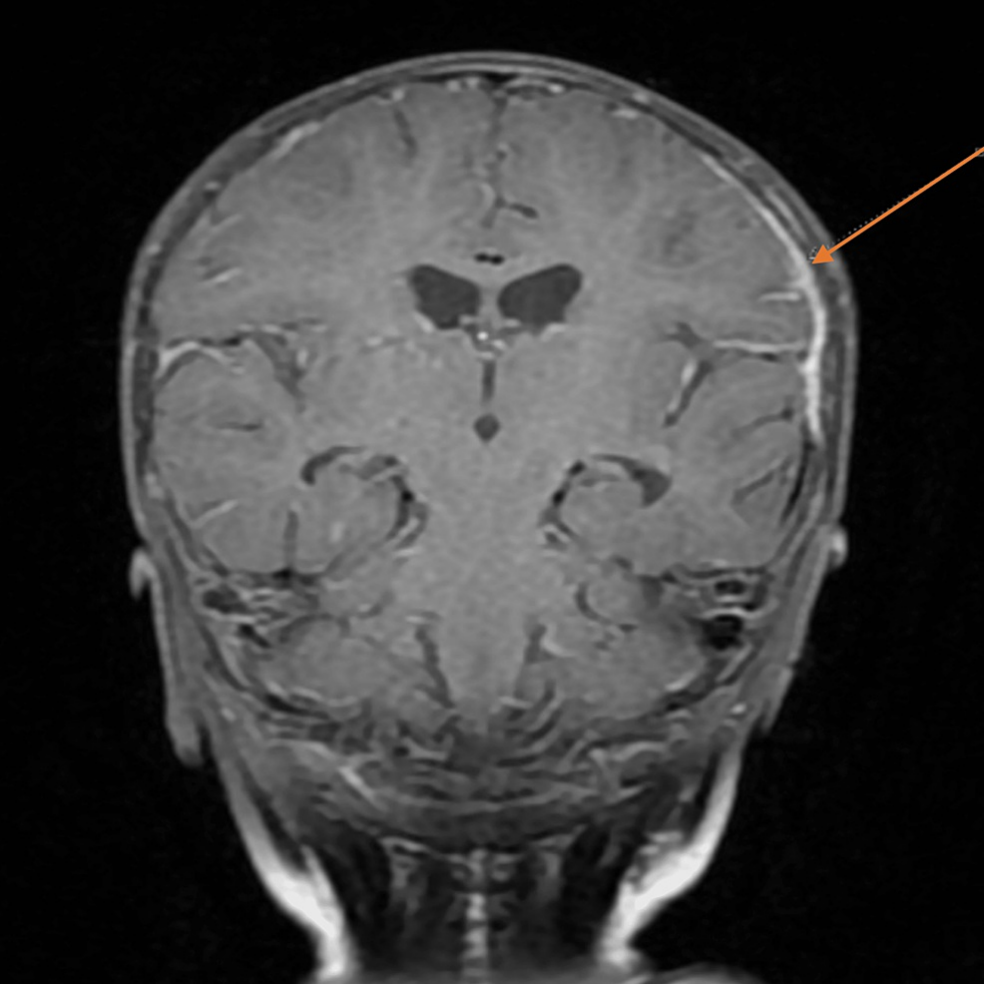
Brain MRI coronal view showing multiple bilateral small intracranial abscesses.
